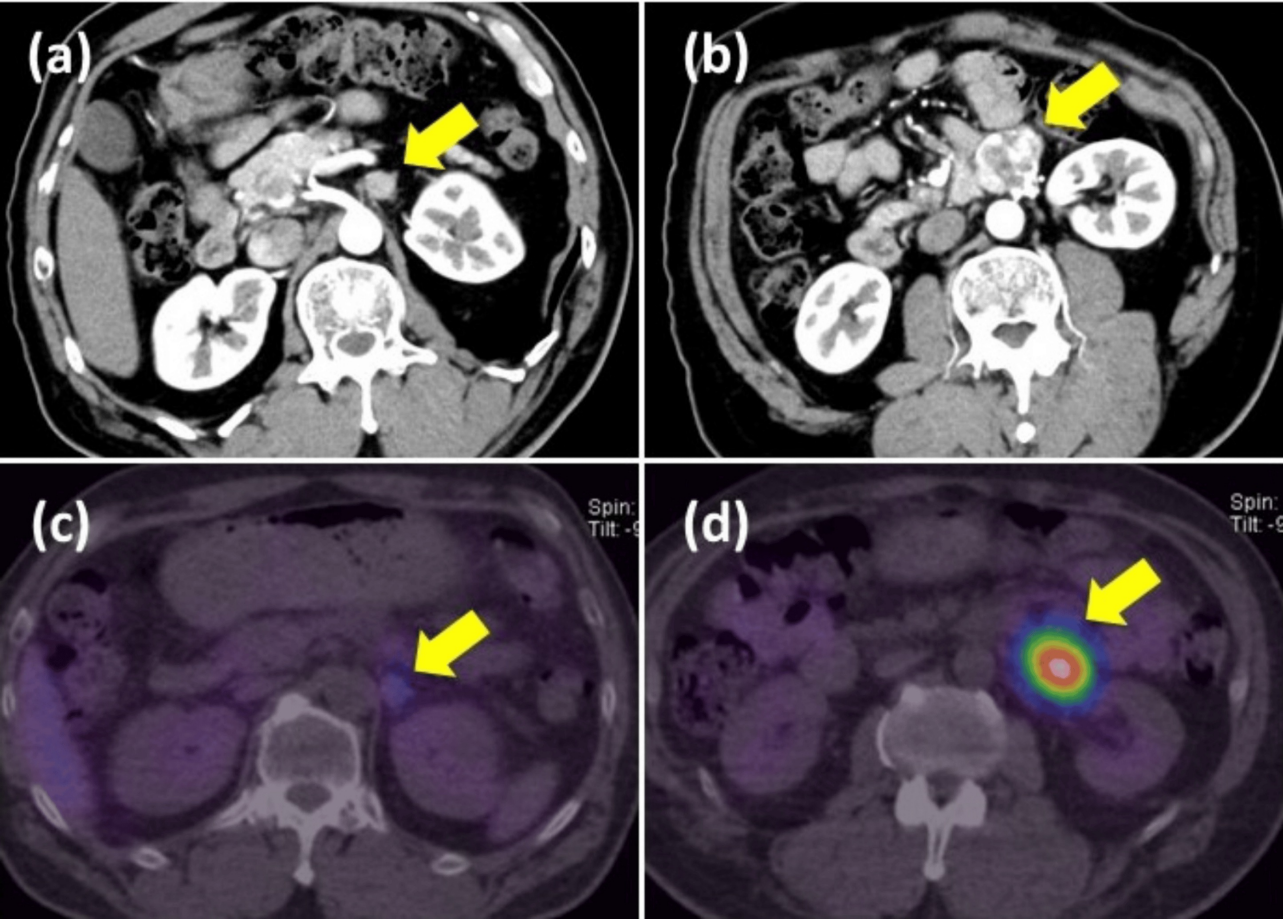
Imaging diagnosis Abdominal enhanced computed tomography revealed a left adrenal tumor (a) and enhanced mass on the left renal hilum (b). Each tumor showed uptake on ^123^I-MIBG scintigraphy (c, d).
